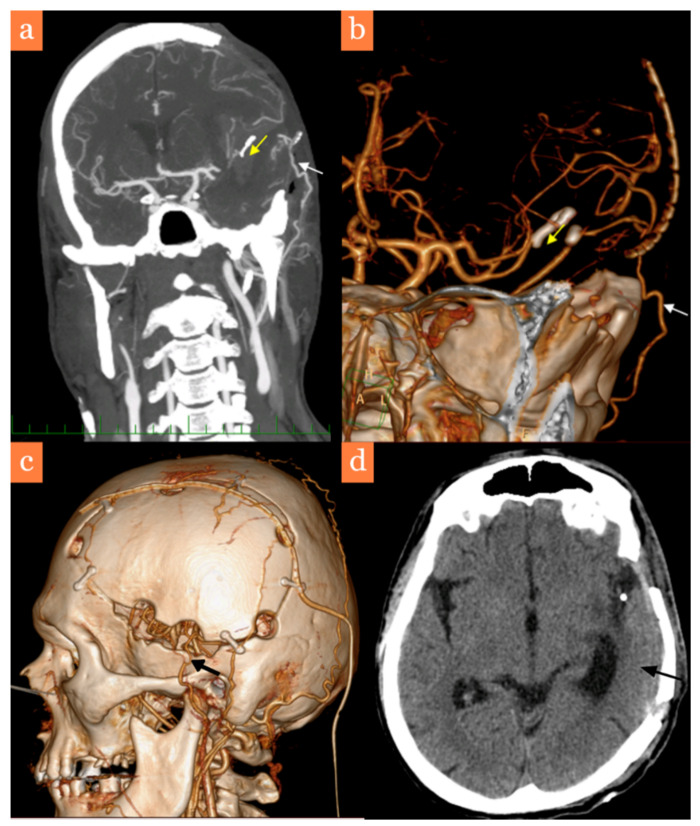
Graphic visualization after the second surgery. (a,b) CTA on the first postoperative day showing the trapped pseudoaneurysm (yellow arrow) and the EC-IC bypass (white arrow), (c) 3D side view after cranioplasty with an autologous bone flap with a CTA demonstrating the patent EC-IC bypass (black arrow), (d) CT after cranioplasty showing resorption of the remaining intracerebral hematoma and the ischemia-free temporal lobe (black arrow).
